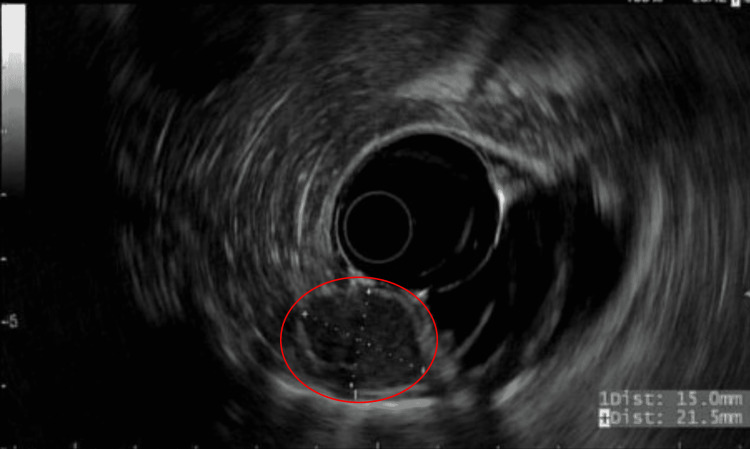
Ultrasound image with hypoechoic lesion measuring 1.5 cm × 2 cm
